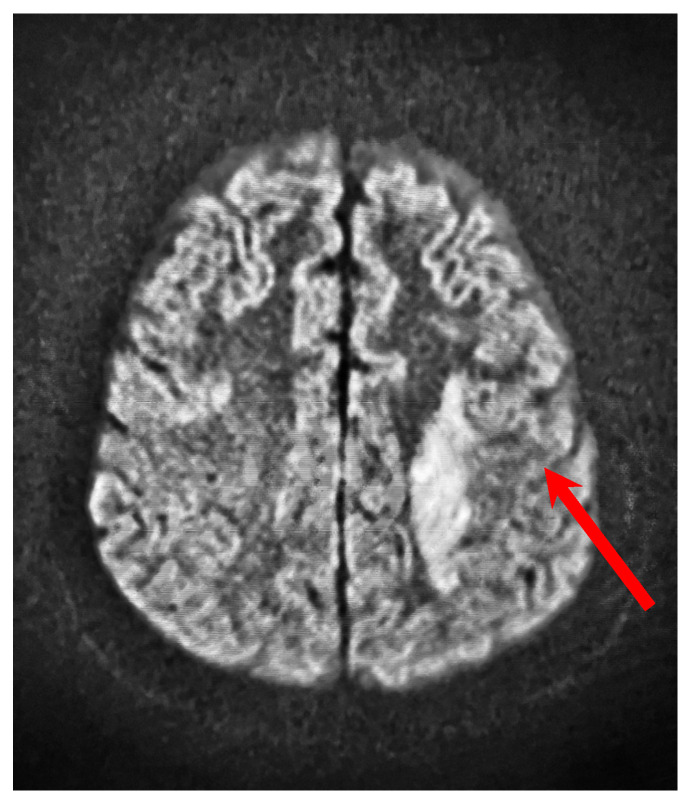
MRI scan of the head—baseline. The red arrow indicates the stroke focus.
